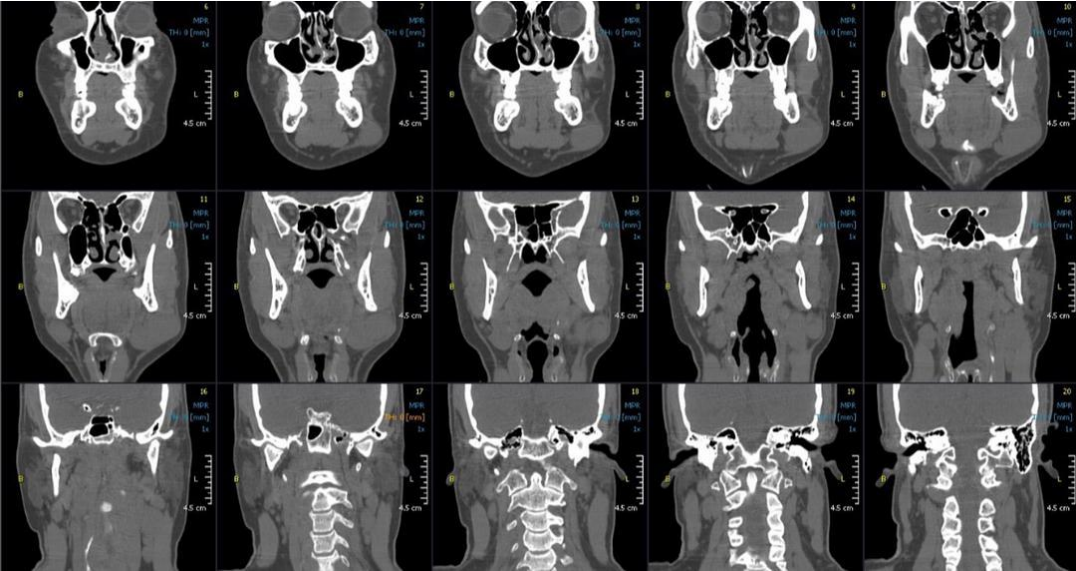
CT scan of patient's head and neck revealing two enhancing radiolucent masses in submandibular spaces of patient, especially in left side
